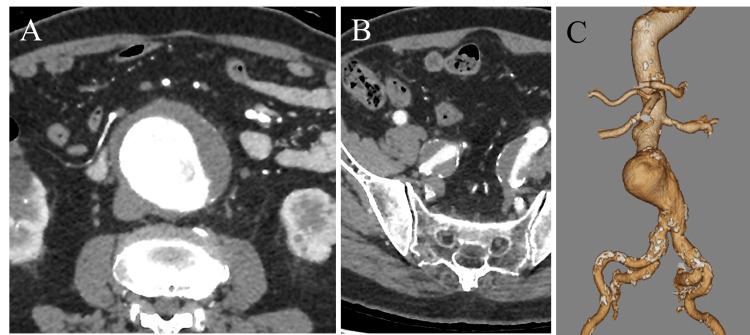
Preoperative images A contrast-enhanced CT showing an AAA measuring 64 mm, a right internal iliac artery aneurysm measuring 28 mm, and a left internal iliac artery aneurysm measuring 22 mm. (A,B) Arterial phase. (C) Volume-rendering image.
